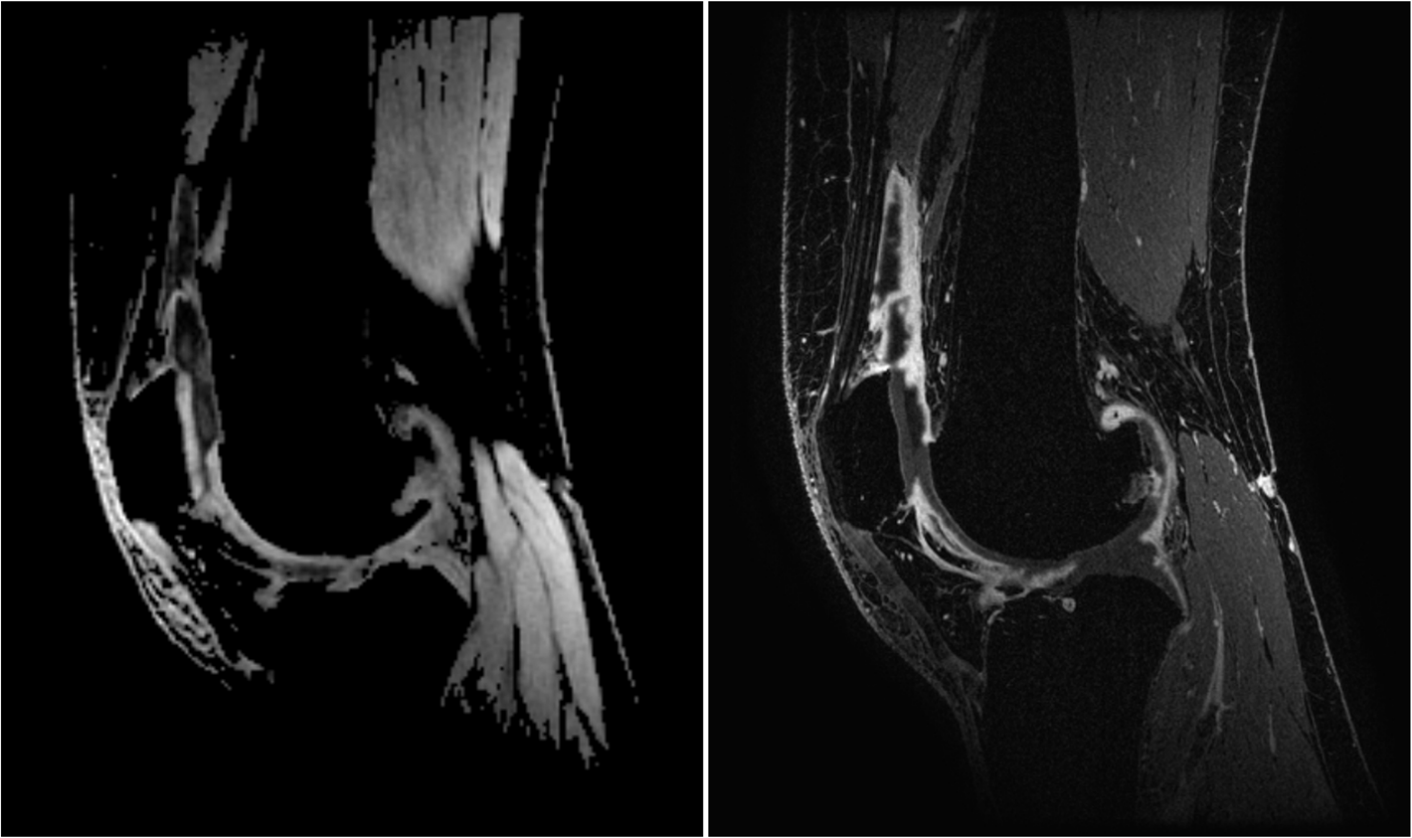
Sagittal qDESS hybrid difference image (left) and CE-MRI (right), both at the level of the patella
